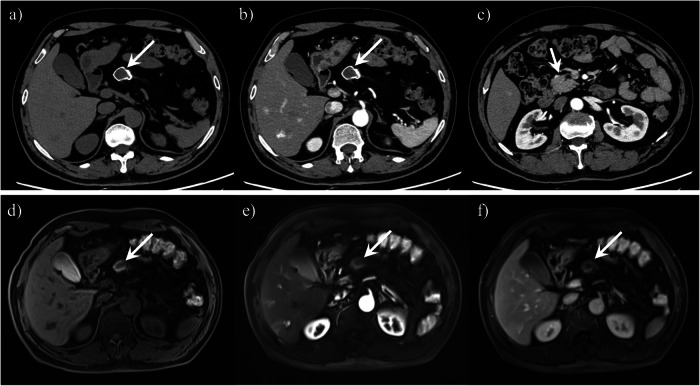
A 62-year-old man presented with dorsal pancreatic agenesis and a pancreatic pseudocyst. Axial unenhanced computed tomography (CT) image (a) showed that the well-defined lesion (arrow) was slightly hypodense with peripheral calcification. Contrast-enhanced CT image (b) showed that the tumor had no enhancement (arrow). Contrast-enhanced CT image (c) showed only the pancreatic uncinate process and head (arrow), but most of the rest of the pancreas was not visualized. The tumor (arrow) showed slightly hypointensity with peripheral hyperintensity on axial T1-weighted fat-suppressed MR image (d). Arterial (e) and venous (f) phase MR images showed that the tumor had no enhancement
